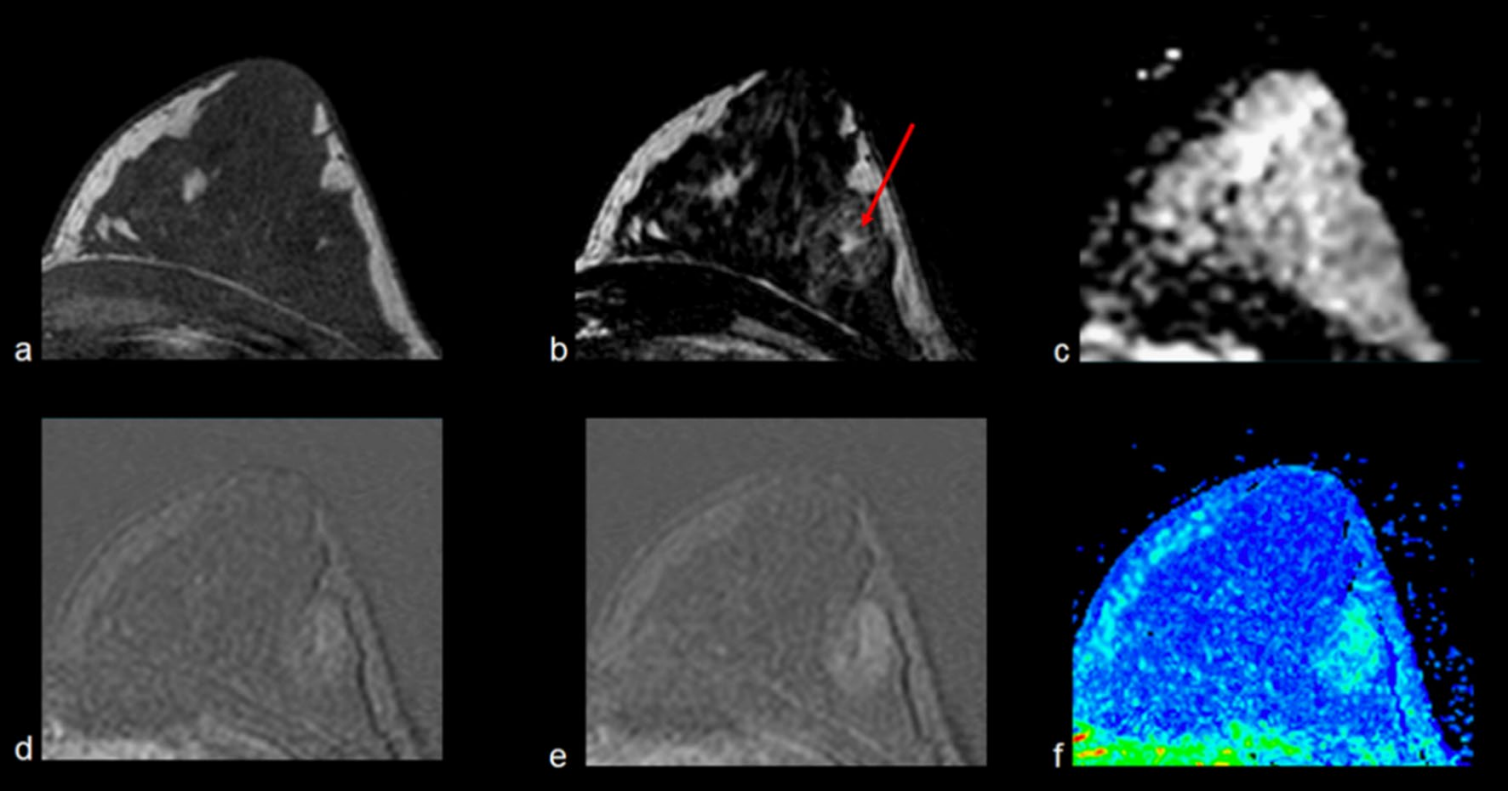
Example of MRI findings of breast lesions categorized as BIRADS 4A by previous echography. A 27-year-old patient with a family history of breast cancer in mother and grandmother. Above: T1-W axial (a), T2 VISTA axial (b) and ADC map (c) show a heterogeneous mass with a solid component in the periphery and a hyperintense central area due to a fatty component, no restricted diffusivity with an ADC of 1.9 × 10^−3^mm^2^/s. Bottom: initial (d) and delayed (e) postcontrast subtractions, and the wash-in map (f) show a progressive uptake of contrast. Morphological and kinetic features are compatible with hamartoma reported in previous echography. It was assigned as the BIRADS-2 category
